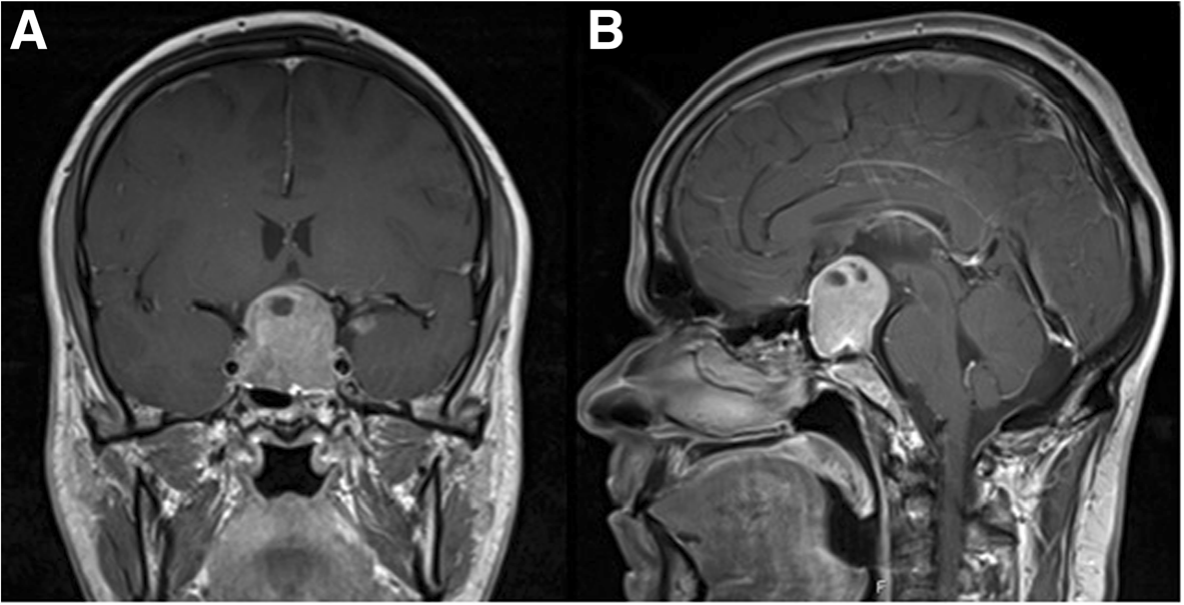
Pituitary magnetic resonance imaging. T1-weighted coronal view (a) and sagittal view (b) post-gadolinium showing a large macroadenoma
